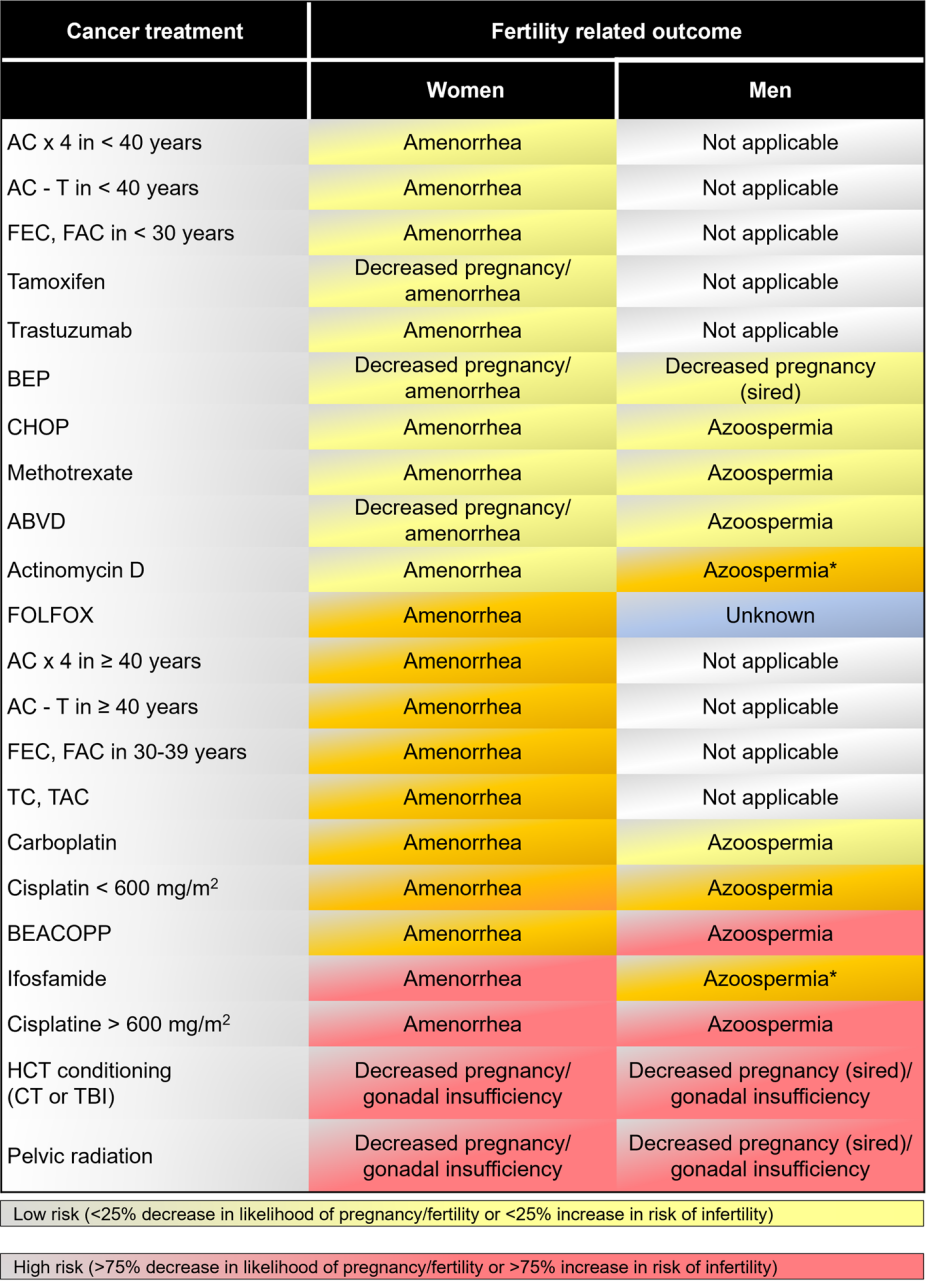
Risk of infertility associated with antineoplastic treatment in adult cancer patients. *Azoospermia likely when given with other highly sterilizing agents. *ABVD* doxorubicin (Adriamycin^®^), bleomycin, vinblastine and dacarbazine; *AC* doxorubicin (Adriamycin^®^) and cyclophosphamide; *AC-T* doxorubicin (Adriamycin^®^), cyclophosphamide and paclitaxel (Taxol^®^); *BEACOPP* bleomycin, etoposide, doxorubicin (Adriamycin^®^), cyclophosphamide, vincristine (Oncovin^®^), procarbazine and prednisone; *BEP* bleomycin, etoposide and platinum; *CHOP* cyclophosphamide, doxorubicin (Adriamycin^®^), vincristine and prednisone; *CT* chemotherapy; *FAC* fluorouracil, doxorubicin (Adriamycin^®^) and cyclophosphamide; *FEC* fluorouracil, epirubicin and cyclophosphamide; *FOLFOX* fluorouracil, leucovorin and oxaliplatin; *HCT* haematopoietic cell transplantation; *TAC* docetaxel (Taxotere^®^), doxorubicin (Adriamycin^®^) and cyclophosphamide; *TBI* total body irradiation; *TC* docetaxel (Taxotere^®^) and cyclophosphamide
